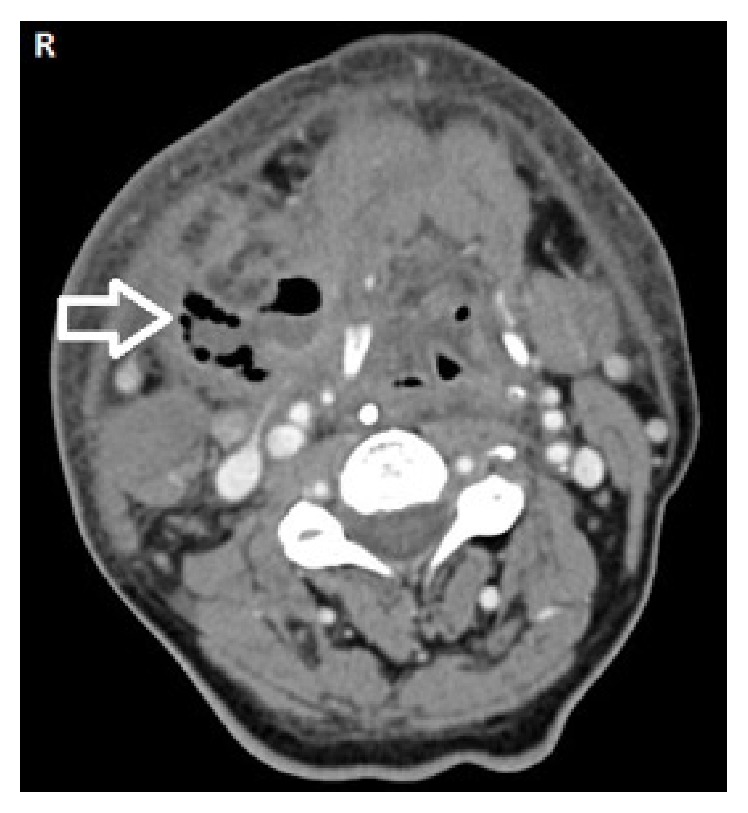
Axial neck CT image showing cervical subcutaneous gas (white arrow).
